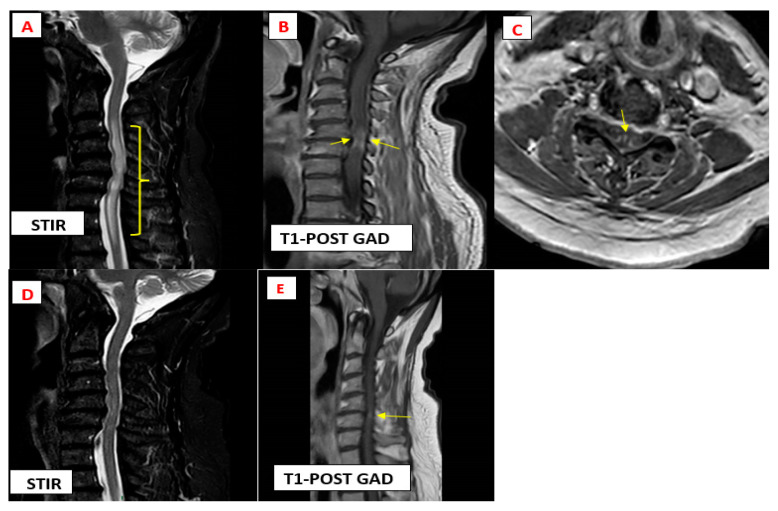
Magnetic resonance imaging findings typical of cervical myelopathy secondary to neurosarocidosis.
